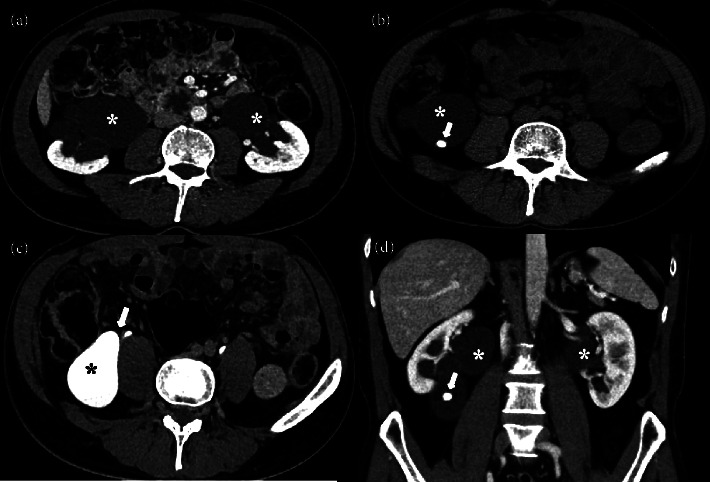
(a) The nephrogram phase of the contrast enhanced CT scan shows severe bilateral dilatation of the renal calyceal system and renal pelvises (^*∗*^). (b) The CT scan shows a severely dilated right kidney pelvis (^*∗*^) with a nonobstructing stone in it (arrow). (c) The urogram phase of the contrast enhanced CT scan shows a severely dilated contrast filled right renal pelvis (^*∗*^) and a normal caliber right renal ureter separated by ureteropelvic junction stricture (arrow). (d) The coronal view of the nephrogram phase of the contrast enhanced CT scan shows bilateral dilatation of renal pelvises (^*∗*^), more severe in the right side, and a nonobstructing stone in the right renal pelvic (arrow).
